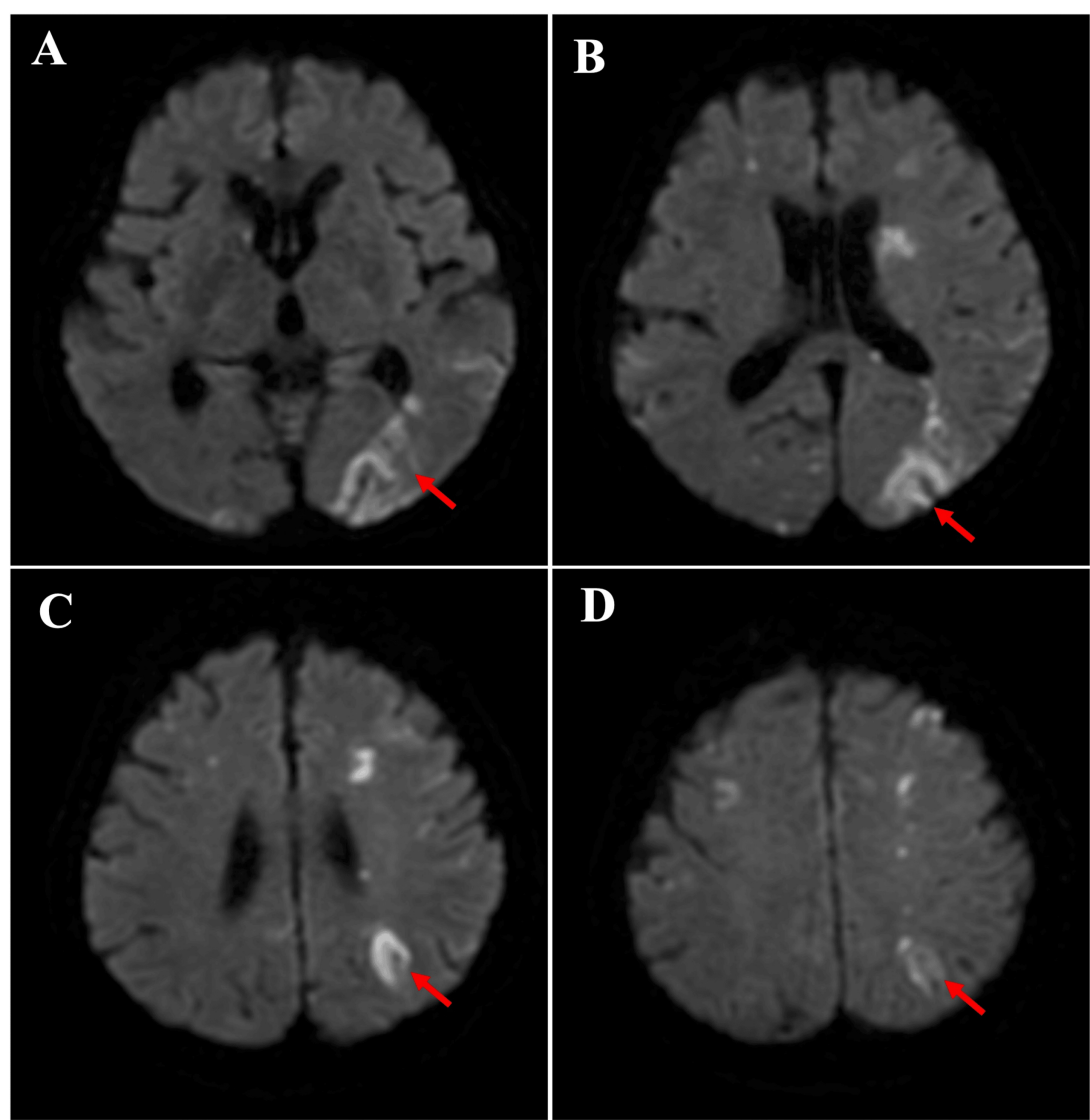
Neuroradiological findings after emergency coil embolization. Magnetic resonance imaging (MRI) of the head revealed acute cerebral infarctions in the anterior and posterior watershed areas and the deep internal border zones (red arrows).
